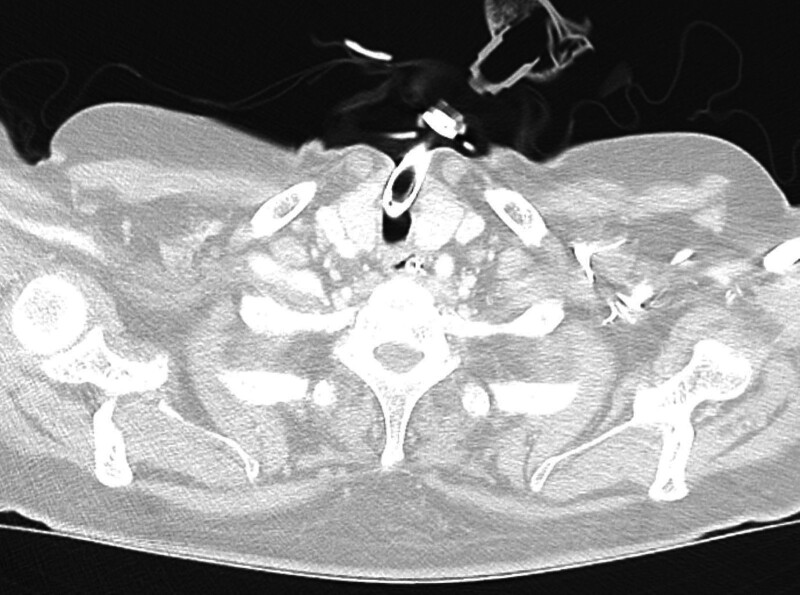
Follow-up axial CT scan at the thoracic inlet level shows resolution of subcutaneous emphysema. No residual air is visible within the soft tissues of the neck or chest wall. The tracheostomy tube remains in place without evidence of peritubal air leakage or re-accumulation. CT = computed tomography.
